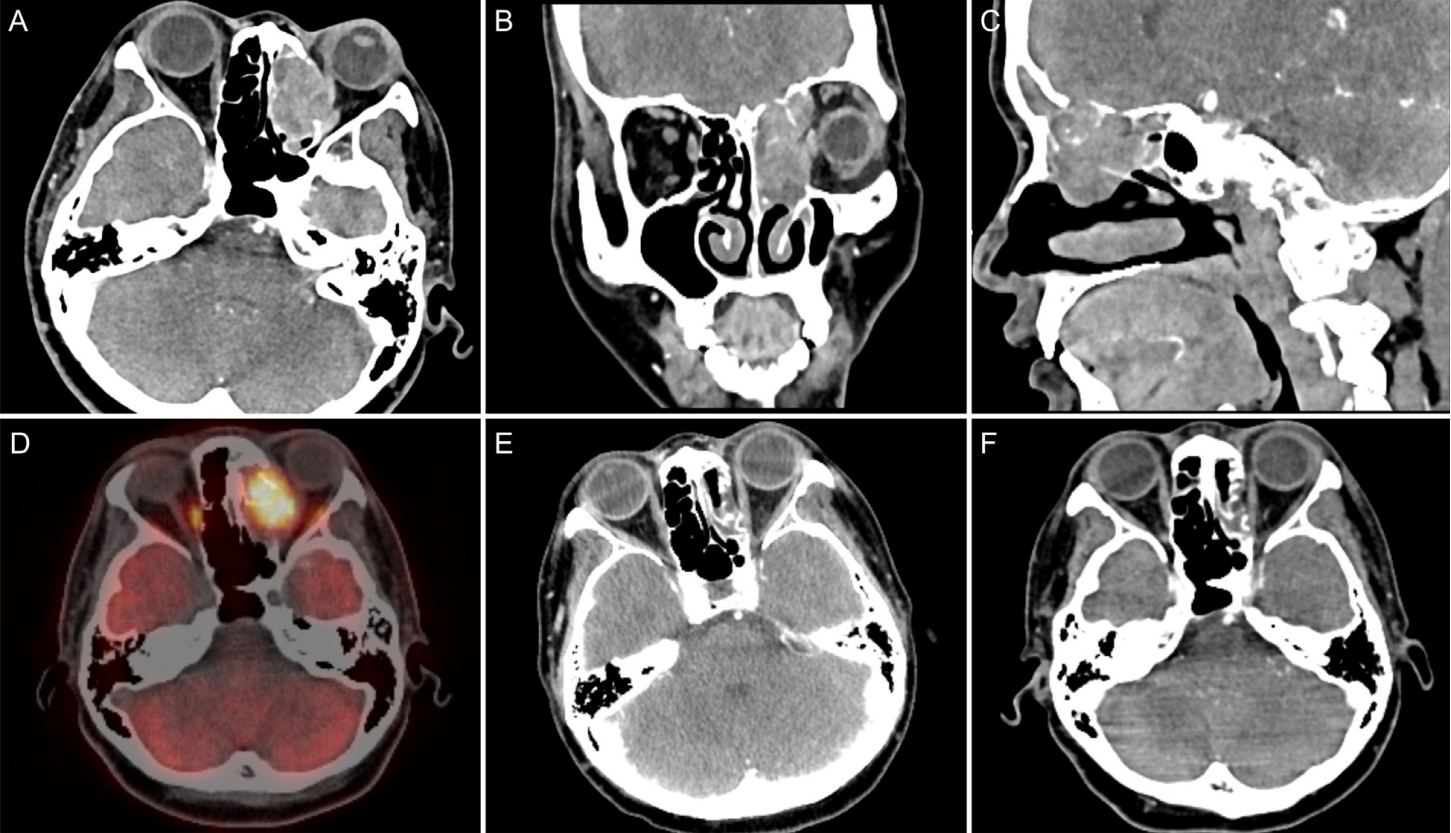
(A) CT axial view showed a mass in the left ethmoid sinus that was poorly demarcated from the left rectus medialis; (B) CT coronal view showed the mass invading the left orbit with intracranial invasion; (C) CT sagittal view shows the mass invading the frontal sinus with intracranial invasion; (D) PET/CT revealed a hypermetabolic mass with an SUVmax of 27.7 in the left ethmoid sinus; (E) CT after three cycles of consolidation chemotherapy revealed that the left ethmoid mass was considerably reduced compared with the previous mass, and the efficacy evaluation was PR; (F) the lesions reached a state of sustained remission after 13 cycles of immunotherapy, and the efficacy evaluation remained a PR.
